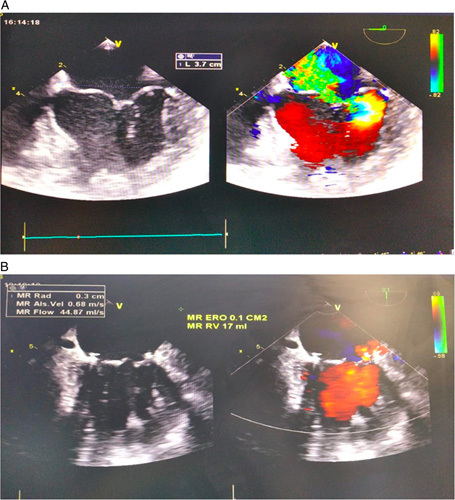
Patient 2 6 months evaluation and post-MV repair echocardiography.
